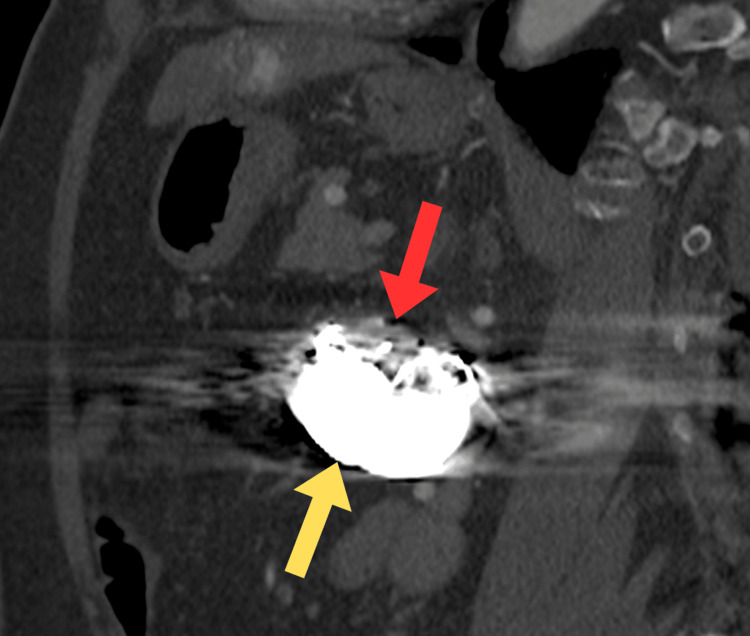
Angio-CT scan during follow-up. The yellow arrow indicates embolization coils. The red arrow indicates the trace filling portion of the aneurysmal sac.
